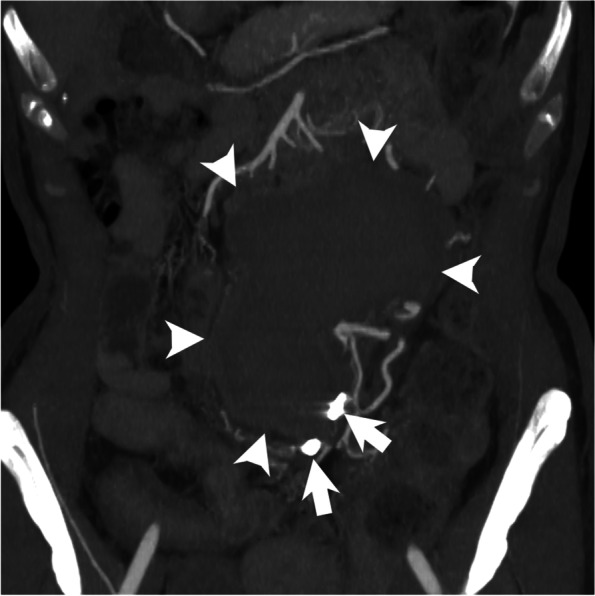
Coronal partial maximum intensity projection image reconstructed from computed tomography angiography obtained 3 months after transcatheter arterial embolization showing the lack of enhancement of the superior rectal artery trunk aneurysm. Note the coils deployed proximally and distally to the aneurysm (arrows) and decreased size of the retroperitoneal hematoma (arrowheads)
